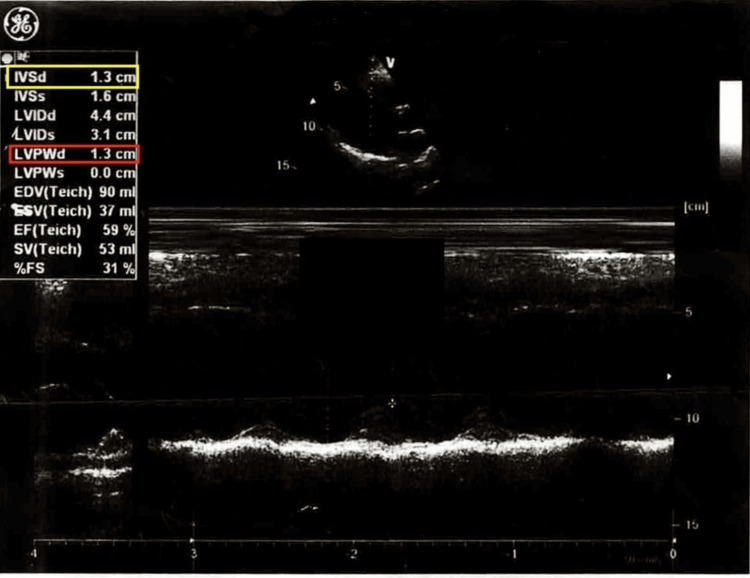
Echocardiography showing left ventricular hypertrophy Yellow rectangle: interventricular septal thickness in diastole (IVSd) of 1.3 cm (normal = 0.6cm - 0.9cm); red rectangle: left ventricular (LV) posterior wall thickness in diastole (LVPWd) of 1.3 cm (normal = 0.6cm - 0.9cm).
